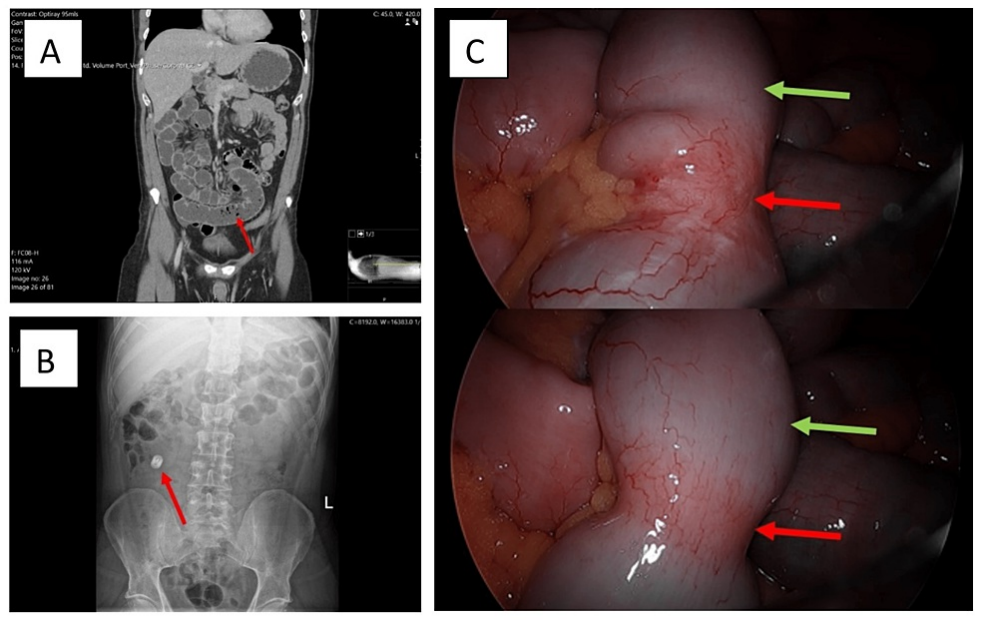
A. Coronal computed tomography of the abdomen scan showing signs of dilated small bowel and fecalization (red arrow), suggesting small bowel obstruction. B. Abdominal X-ray showing the patency capsule that did not pass through the small bowel (red arrow). C. Laparoscopy images showing macroscopic changes in the small bowel. Strictures (red arrows) and dilations (green arrows) are visible in both images.
